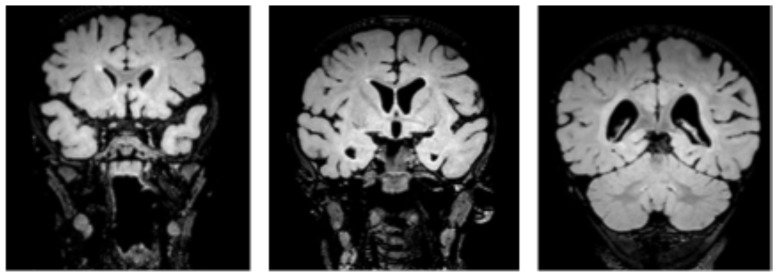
Brain MRI scan of the patient showing a quantitative reduction in bihemispheric white matter, particularly in the posterior regions, with T2/FLAIR hyperintensity observed in the corona radiata and supratrigonal/peritrigonal areas, partly associated with mild ectasia of the perivascular spaces, possibly due to gliosis. The supratentorial ventricular system appears larger than normal, with asymmetric lateral ventricles due to a greater size of the left ventricle and predominance of the posterior sections.
